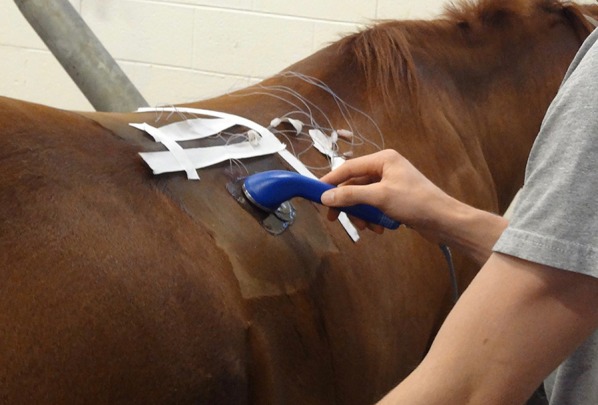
Performing the ultrasound using standard ultrasound transmission gel, for 10 min via a 1.0 MHz sound head with an effective radiating area of 10 cm^2^.
